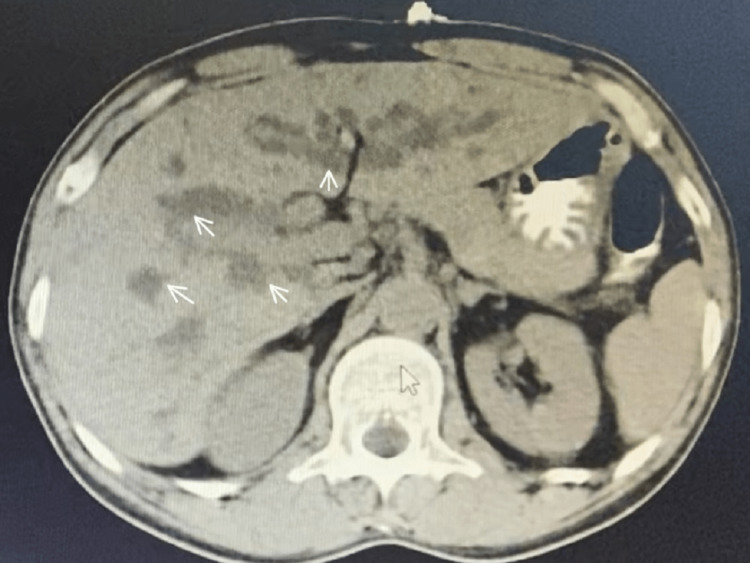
CECT scan of the abdomen CECT: Contrast-enhanced computed tomography, IHBR: Intrahepatic biliary radicals White arrows represent the grossly dilated IHBR
